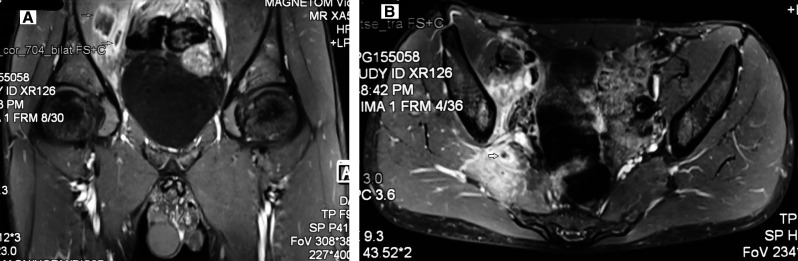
(A) Magnetic resonance imaging of the bilateral hip joints showing collection in the right iliopsoas muscle with surrounding edema (arrows = coronal section). (B) Magnetic resonance imaging of the bilateral hip joints showing collection in the iliopsoas muscle with surrounding edema (arrows = transverse section).
